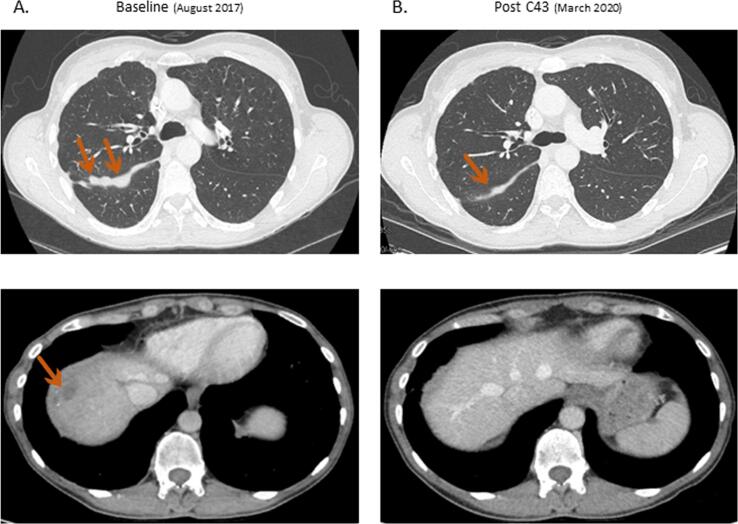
Contrast-enhanced computed tomography (CT) of the ovarian cancer lesions evolution. (A) At baseline, before starting the trastuzumab - pertuzumab therapy, right pleural thickening and one liver lesion. (B) After 43 trastuzumab – pertuzumab cycles (C), partial response with no liver lesion visible and right pleural thickness reduction.
